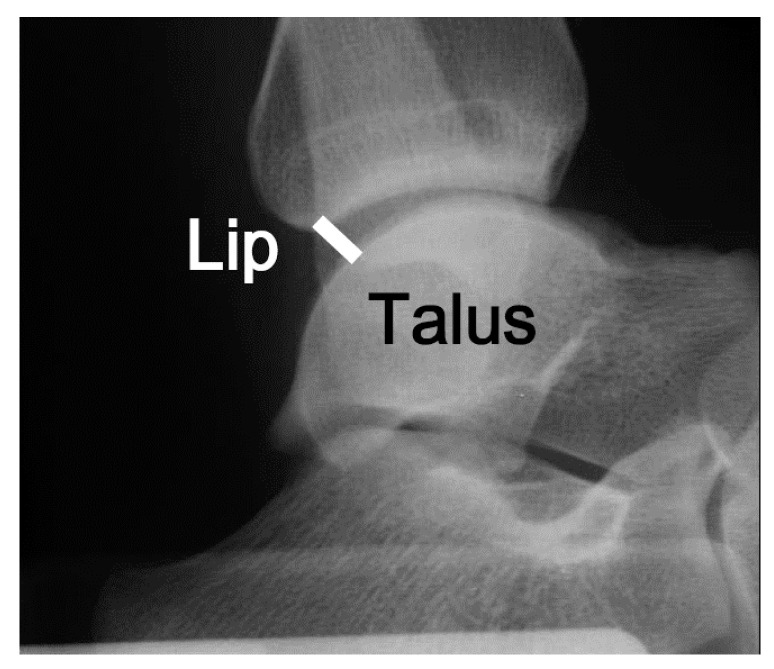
The distance between the tibia and the talus was measured during the X-ray imaging test. The white solid line represents the shortest distance between the tibia and talus. ‘Lip’ indicates the posterior lip of the tibia.
